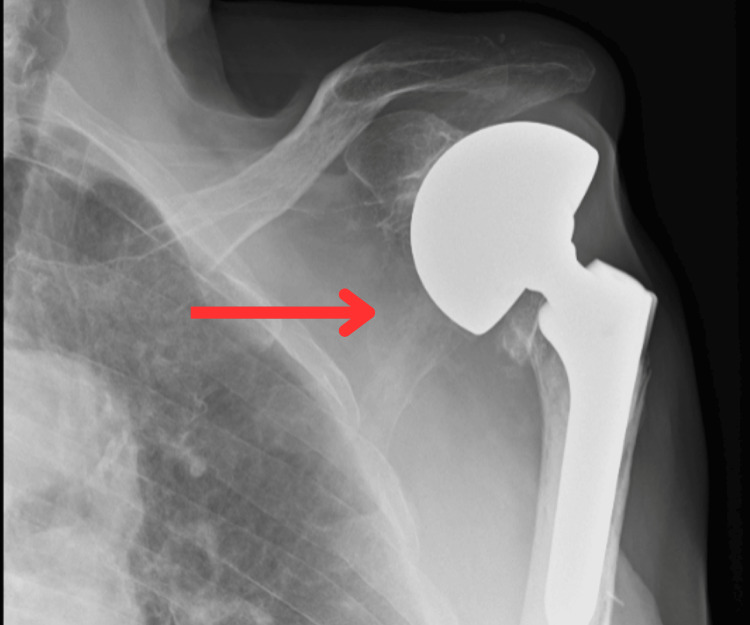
Additional post-operative radiographic views of the left shoulder bipolar hemiarthroplasty conversion
